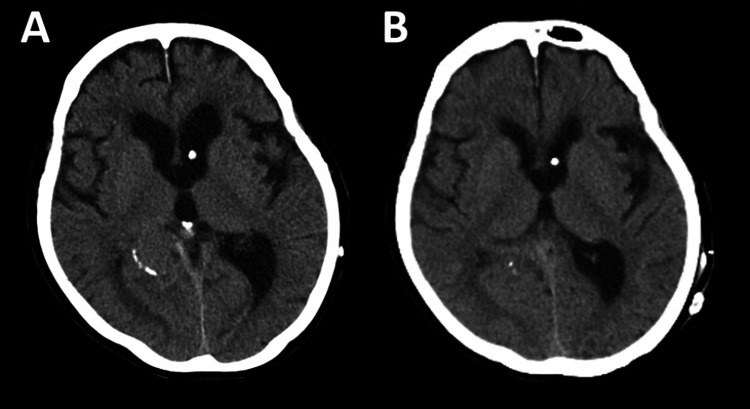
CT images (A) Preoperative CT. (B) Postoperative CT.
